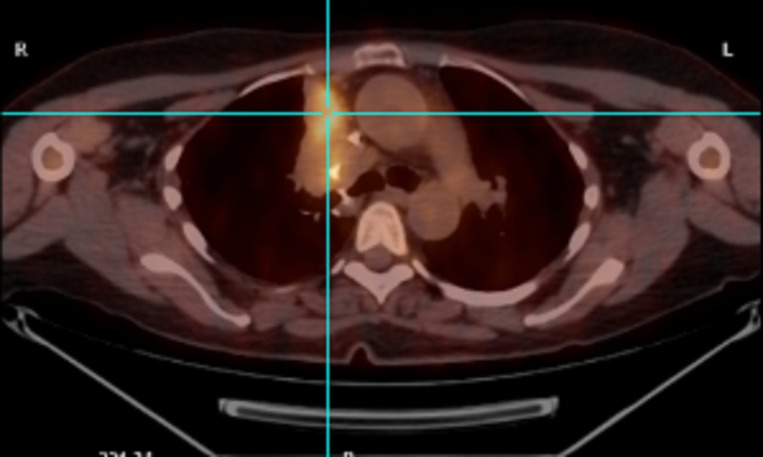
PET/CT scan in May 2016 Pathologic uptake seen within the enlarged right paramediastinal soft tissue mass.
